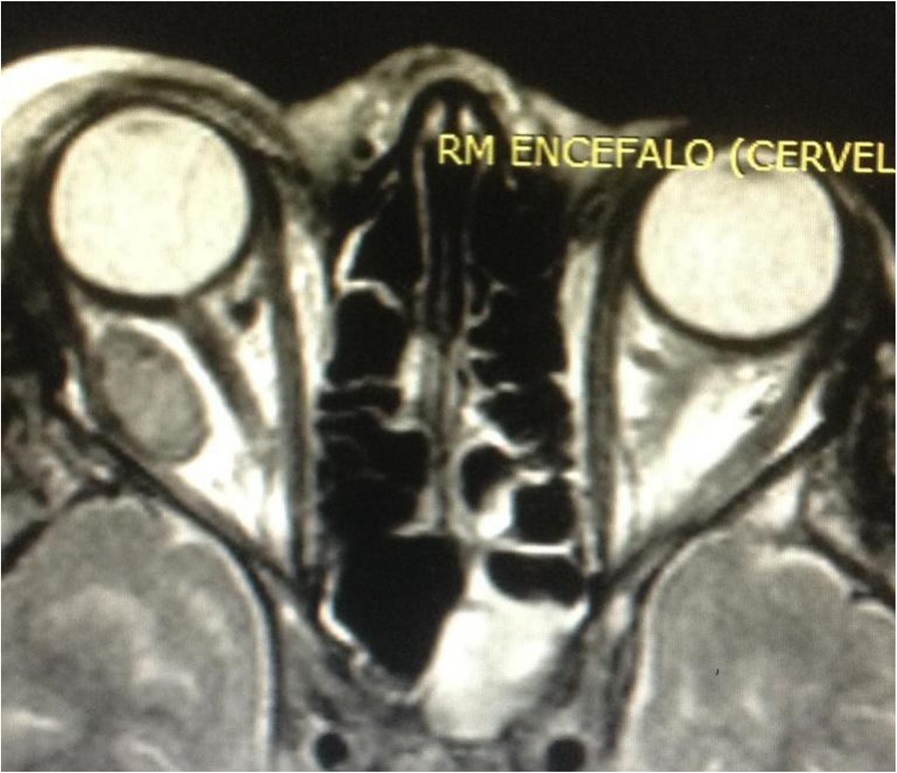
Preoperative MR imaging (axial view): right intraorbital intraconic abscess with signs of left sphenoiditis
